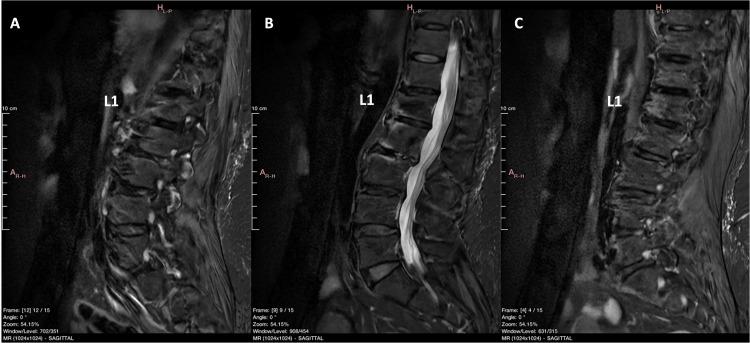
MRI STIR FSE images (sagittal view) of the lumbar spine obtained at 10 months following the two balloons kyphoplasty. No gross signs of a malignant tumor at the L1 vertebra, and/or breaching of the vertebral cortex are apparent. A: left part of the spinal column, B: medial part of the spinal column, C: right part of the spinal column STIR: short tau inversion recovery; FSE: fast spin echo
